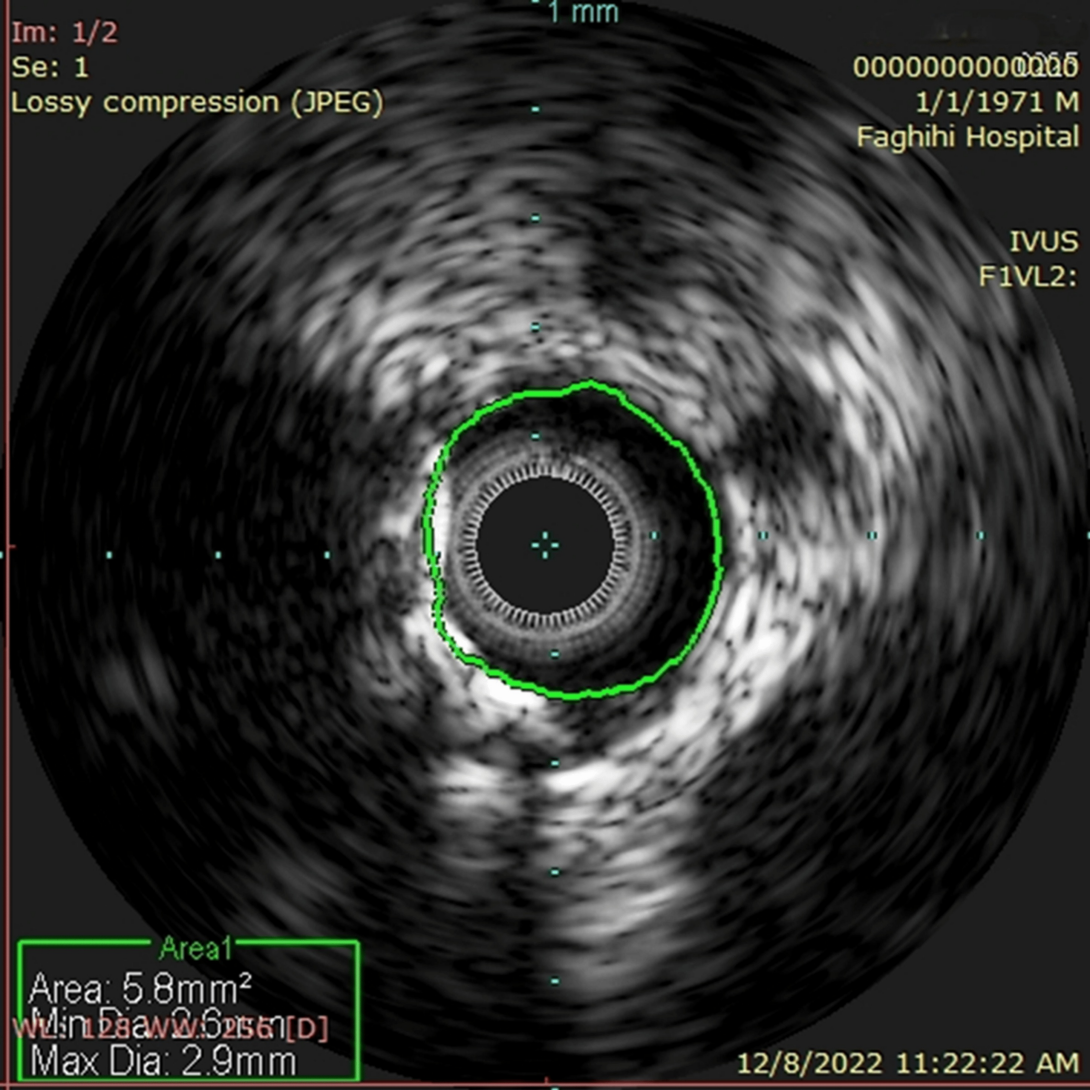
Intravascular ultrasound minimum luminal area (IVUS) assessment picture from one of the studied patients
